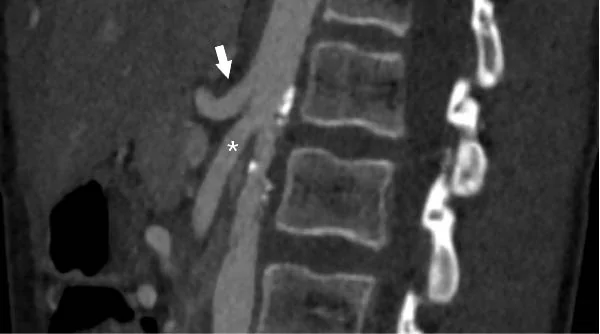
CT angiography, lateral view—neither celiac trunk (arrow) nor SMA (asterisk) showing visible signs of severe stenosis.
